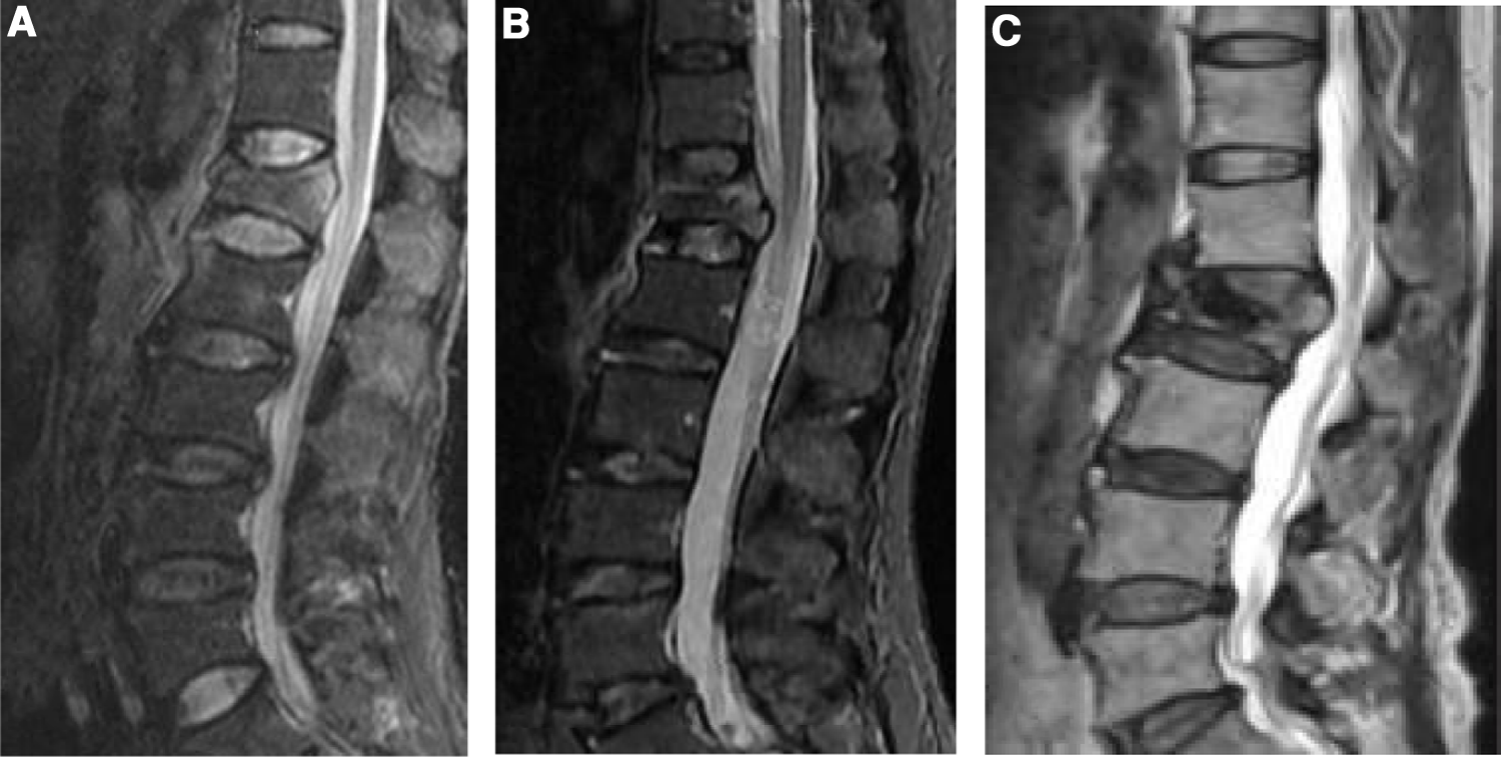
According to the imaging characteristics of disc injury, it was divided into three categories: no injury, mild injury, and moderate-to-severe injury (sagittal MRI images of the thoracolumbar segment before treatment). (A) No intervertebral disc injury (0 points). (B) Mild intervertebral disc injury, signal change, no endplate injury, with or without space change (1 point). (C) Moderate-to-severe intervertebral disc injury, significant signal change, end plate fracture, intervertebral disc contents herniated into vertebral body, intervertebral space change (2 points).
